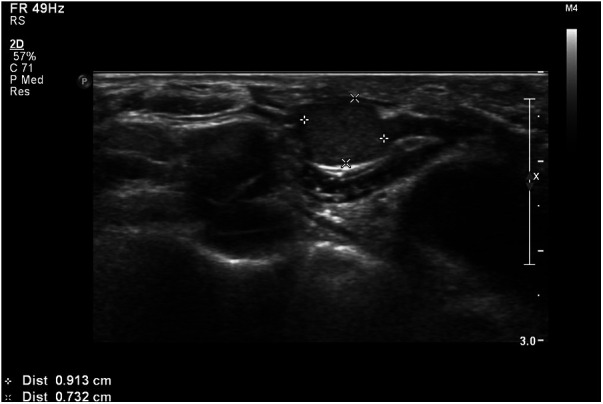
An ultrasound transverse view of a metastatic hypoechoic nodule, measuring 0.9 cm × 1.2 cm in size, within a lymph node between the right sternocleidomastoid and sternohyoid muscles (LNSS).
